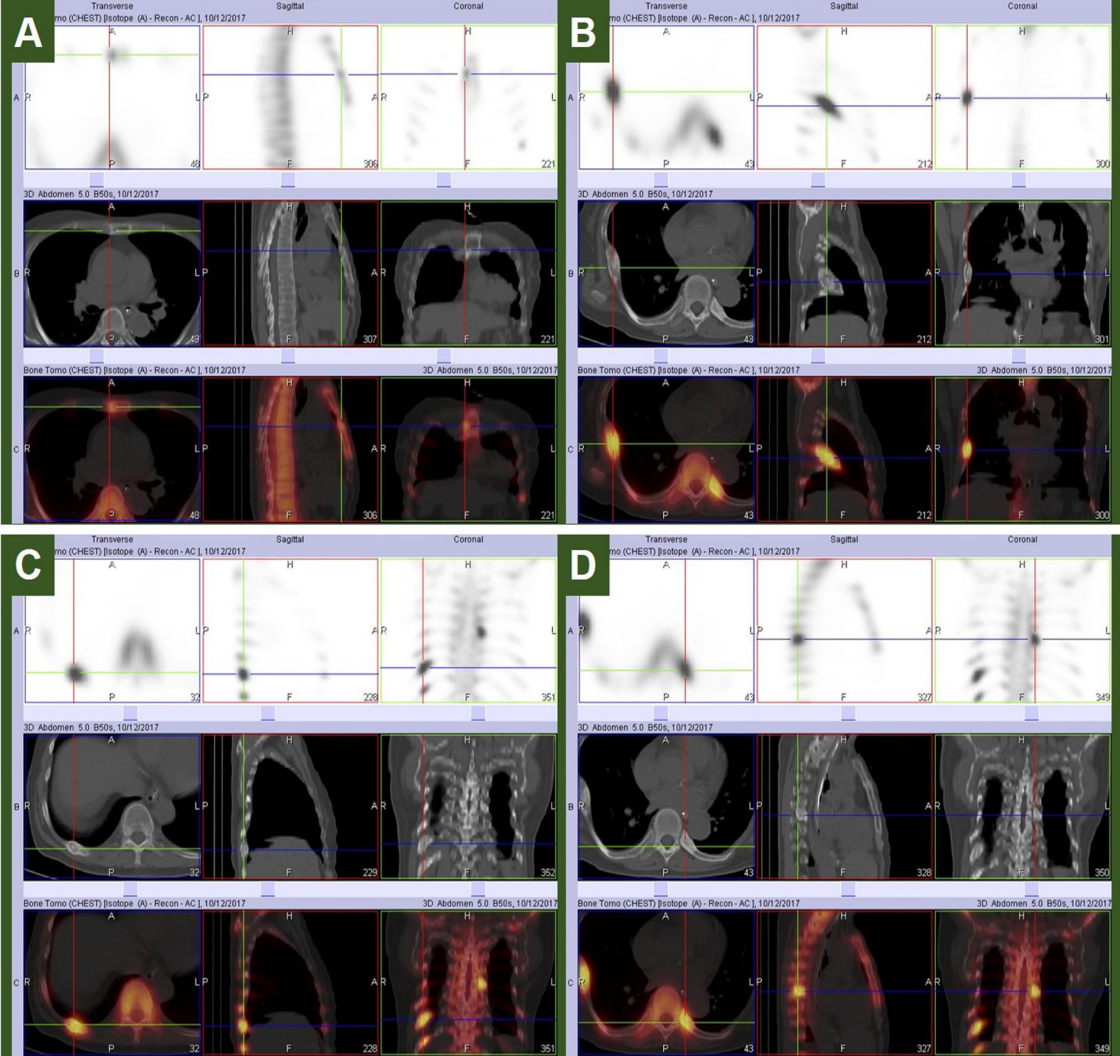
SPECT/CT revealed multiple MDP-avid metastatic foci: (A) mild focus on the right side of the sternum showing mild bone erosion; (B) soft tissue mass on the 7^th^ right rib that was also sestamibi-avid on parathyroid scan; (C) posterior aspect on the 10^th^ right rib showing another soft tissue mass; and (D) 8^th^ left costovertebral junction
